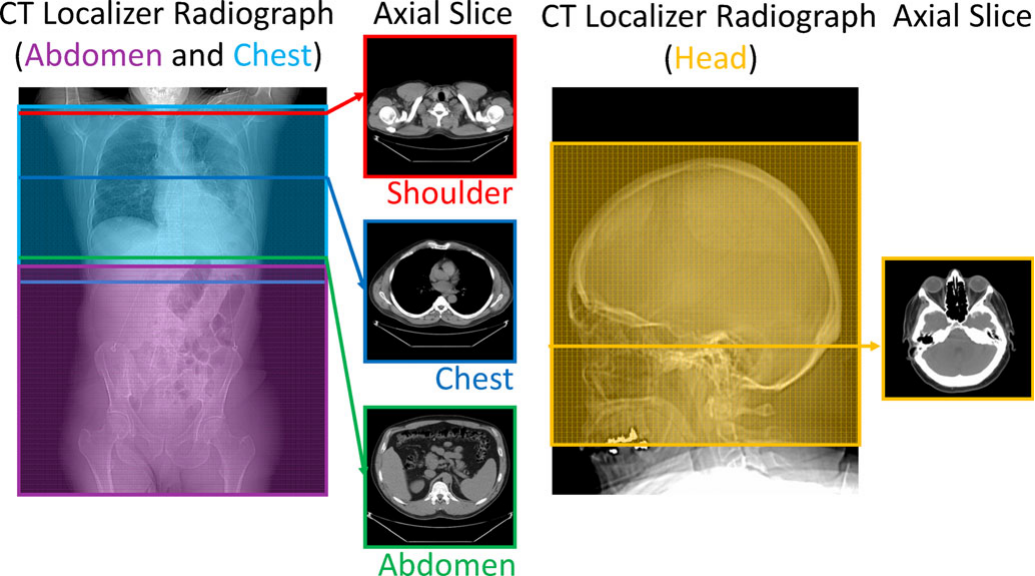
Scan range of adult and pediatric abdomen pelvis (purple), adult chest (cyan), and adult head (orange) scans shown on a CT localizer radiograph. The adult chest scan is broken up into three subsets to produce a total of six datasets. Examples of single axial CT slices of these subsets for shoulders (red), thorax (blue), and abdomen only (green) are shown as well as an example head axial slice (orange).
